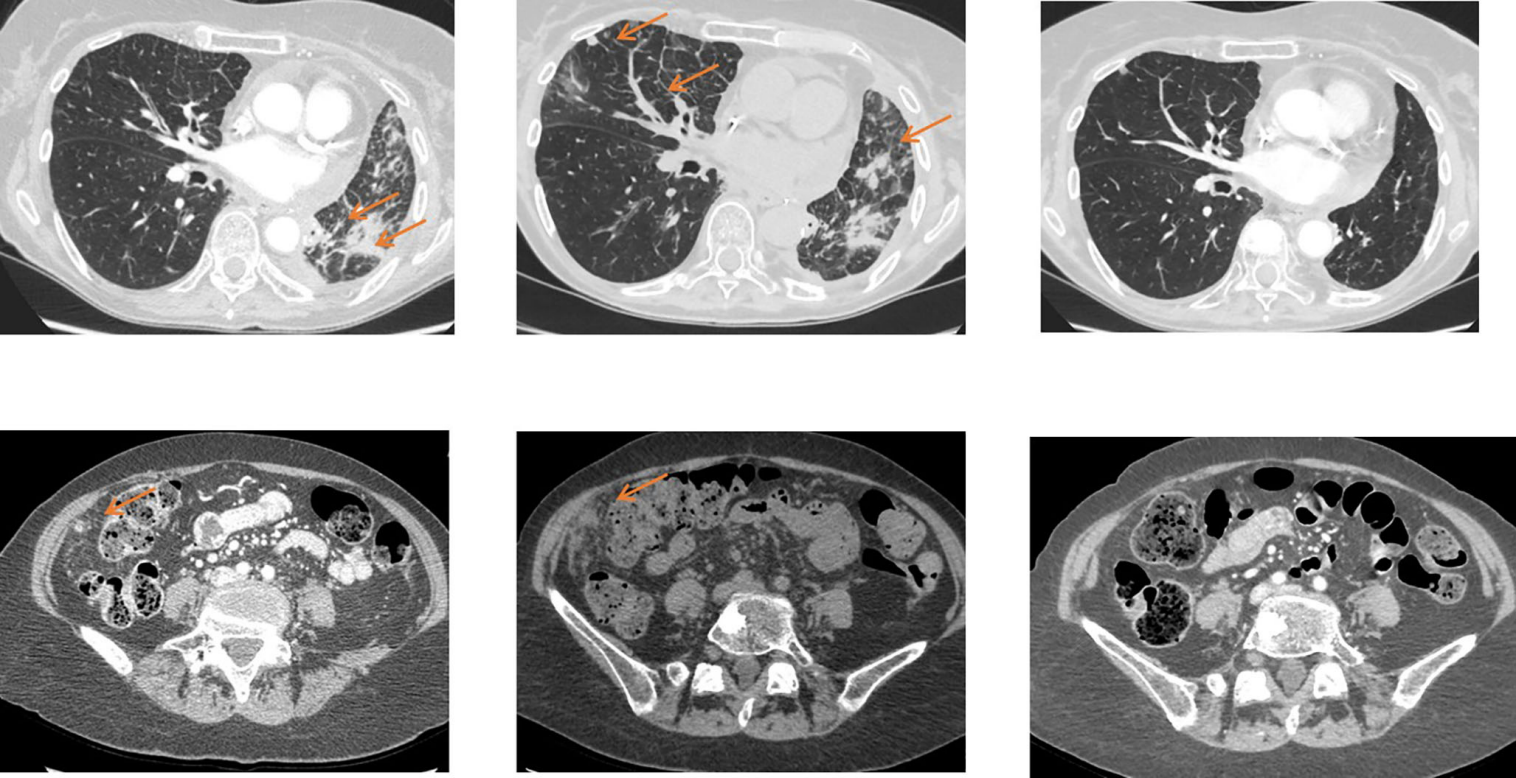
Pseudoprogression on immunotherapy in a 56 yo patient with metastatic non-small cell lung cancer. The baseline image (left) shows lung and peritoneal nodules (arrows). After 4 wks of antiPDL1 therapy (middle), CT shows an increase in previous lesions and the appearance of new lung nodules. Disease was considered immune unconfirmed progressive disease. Six weeks later (right) a dramatic response in all previous lesions was seen classifying the patients as a complete responder and endorsing an earlier diagnosis of pseudoprogression.
